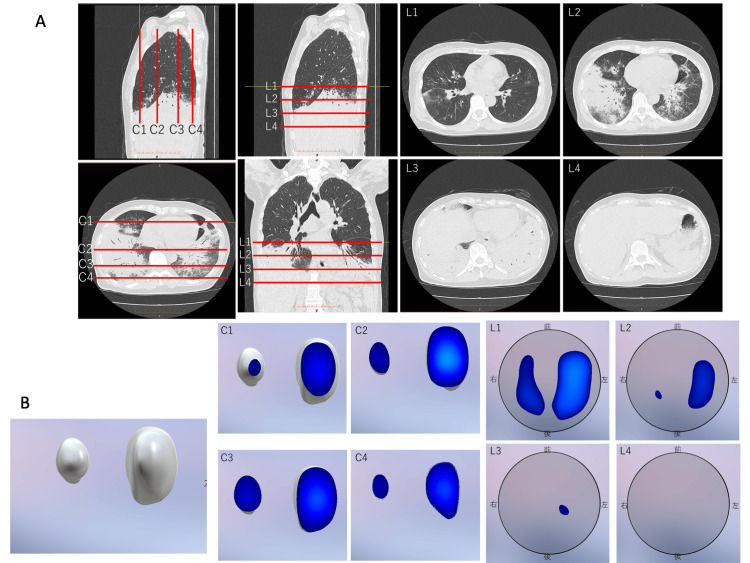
(A) Chest CT of the patient; (B) Chest EIT of the patient. L1 to L4 indicates the position of the scanned layer of EIT. C1 to C4 indicates the coronal section of the EIT scan. EIT: electrical impedance tomography
